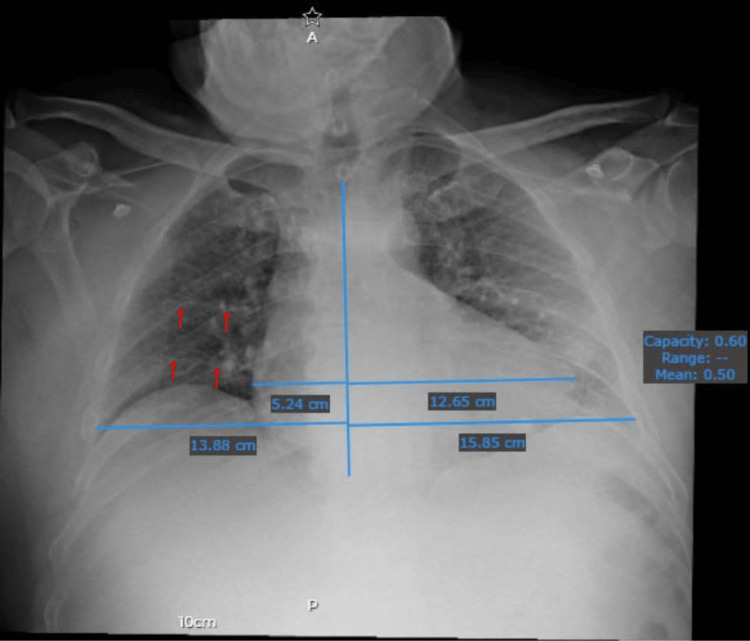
Anteroposterior Chest Radiograph The image reveals a pattern of bilateral alveolar infiltrates, most prominent in the perihilar regions, suggestive of a "butterfly wing" distribution. Linear opacities, identified as Kerley B lines (marked with red arrows), are observed in the lower lung fields, consistent with interlobular septal thickening. The cardiothoracic ratio was assessed using heart and chest widths, as indicated by blue lines: heart width, 17.89 cm; chest width, 29.73 cm. The calculated cardiothoracic ratio (CTR) is approximately 0.60 (17.89 cm / 29.73 cm), exceeding the threshold of 0.50, indicative of cardiomegaly.
